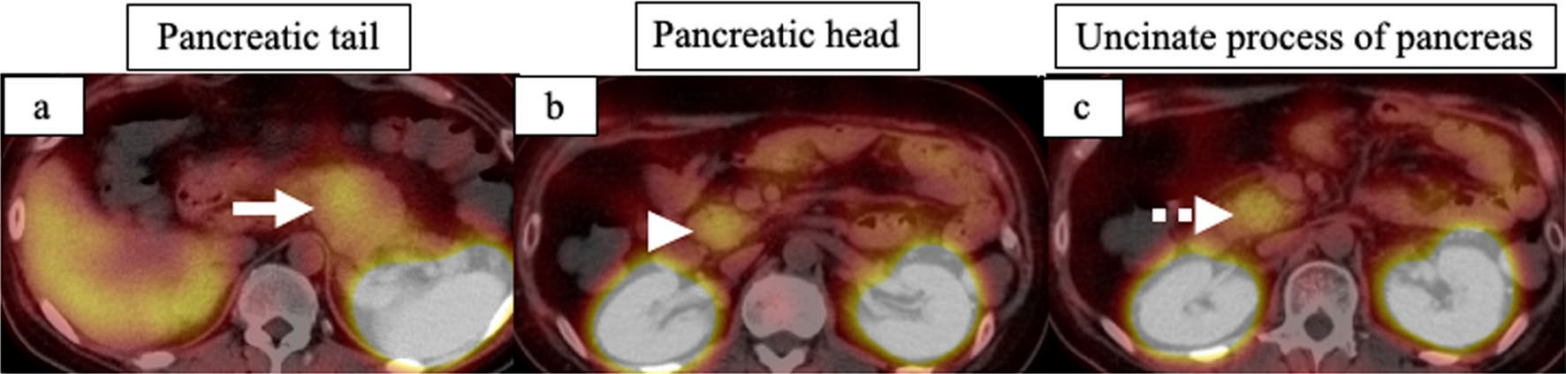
SRS. a SRS reveals a high accumulation of radioactive tracer in the pancreatic tail (white arrow). b SRS reveals a high accumulation of radioactive tracer in the pancreatic head (white arrowhead). c SRS reveals a high accumulation of radioactive tracer in the uncinate process of the pancreas (dotted white arrow). *SRS* somatostatin receptor scintigraphy
